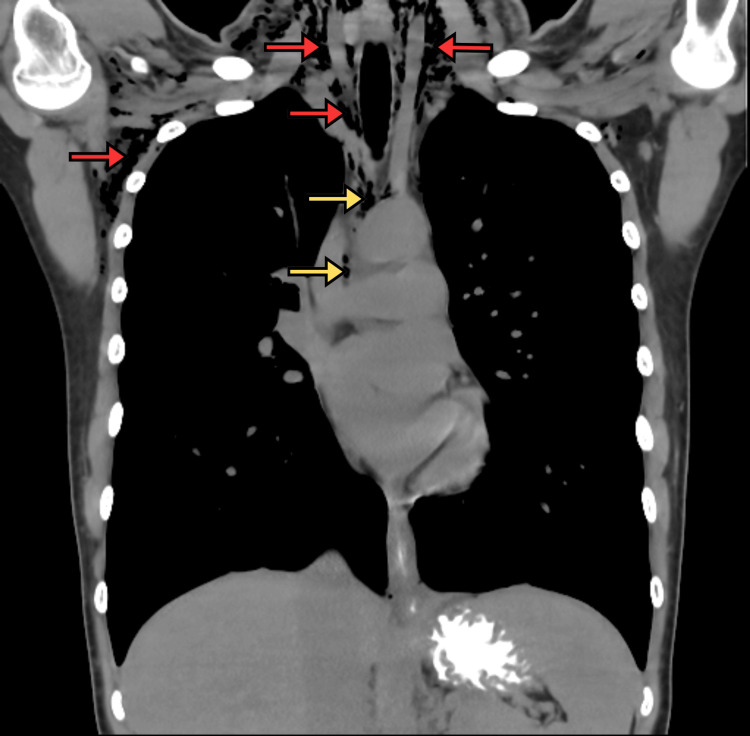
CT chest showing subcutaneous emphysema in the soft tissues of the neck and axilla, with pneumomediastinum Coronal CT imaging of the thorax reveals extensive subcutaneous emphysema, visible as radiolucent air pockets (red arrows) within the cervical, supraclavicular, and axillary soft tissues. Air is also observed within the mediastinum (yellow arrows), consistent with pneumomediastinum.
